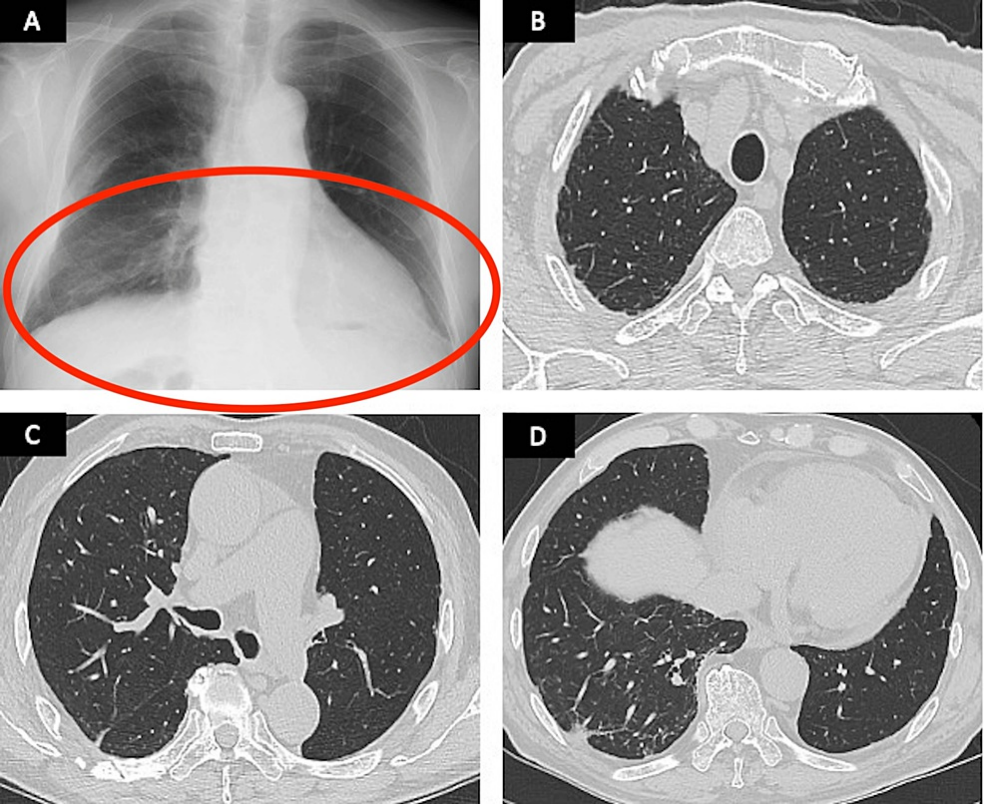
Imaging examination one year after the start of treatment (A) Chest radiograph showing mild reticular shadowing in bilateral lower zones. (B–D) Chest CT showing no recurrence of lung cancer and improvement of interstitial pneumonia. CT, computed tomography
